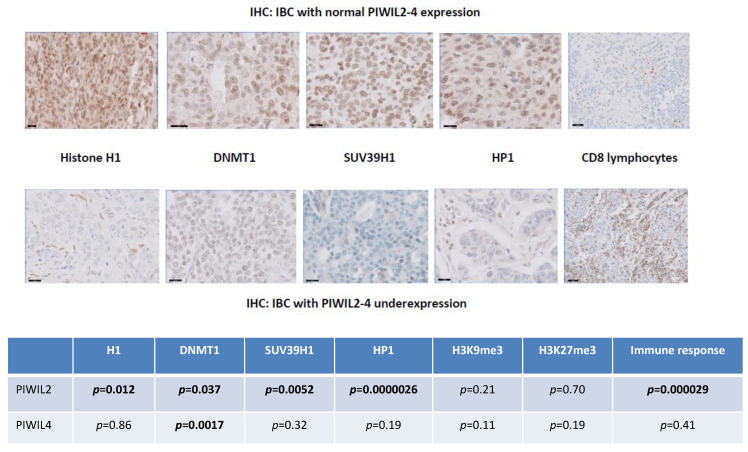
PIWIL2-4 underexpression is correlated with downregulation of H1, DNMT1, HP1 and SUV39H1 and upregulation of CD8 cytotoxic immune response. At magnification ×800.
